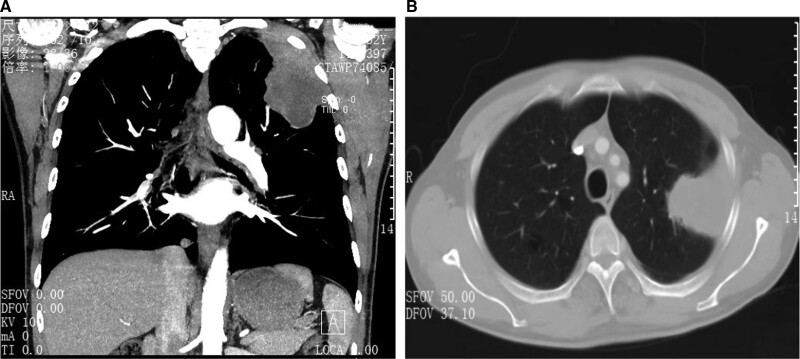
Chest computed tomography demonstrating the tumor in the left upper lobe of the lung invading the chest wall and 3^rd^ to 5^th^ ribs. (A) Coronal section (B) Transverse section.
